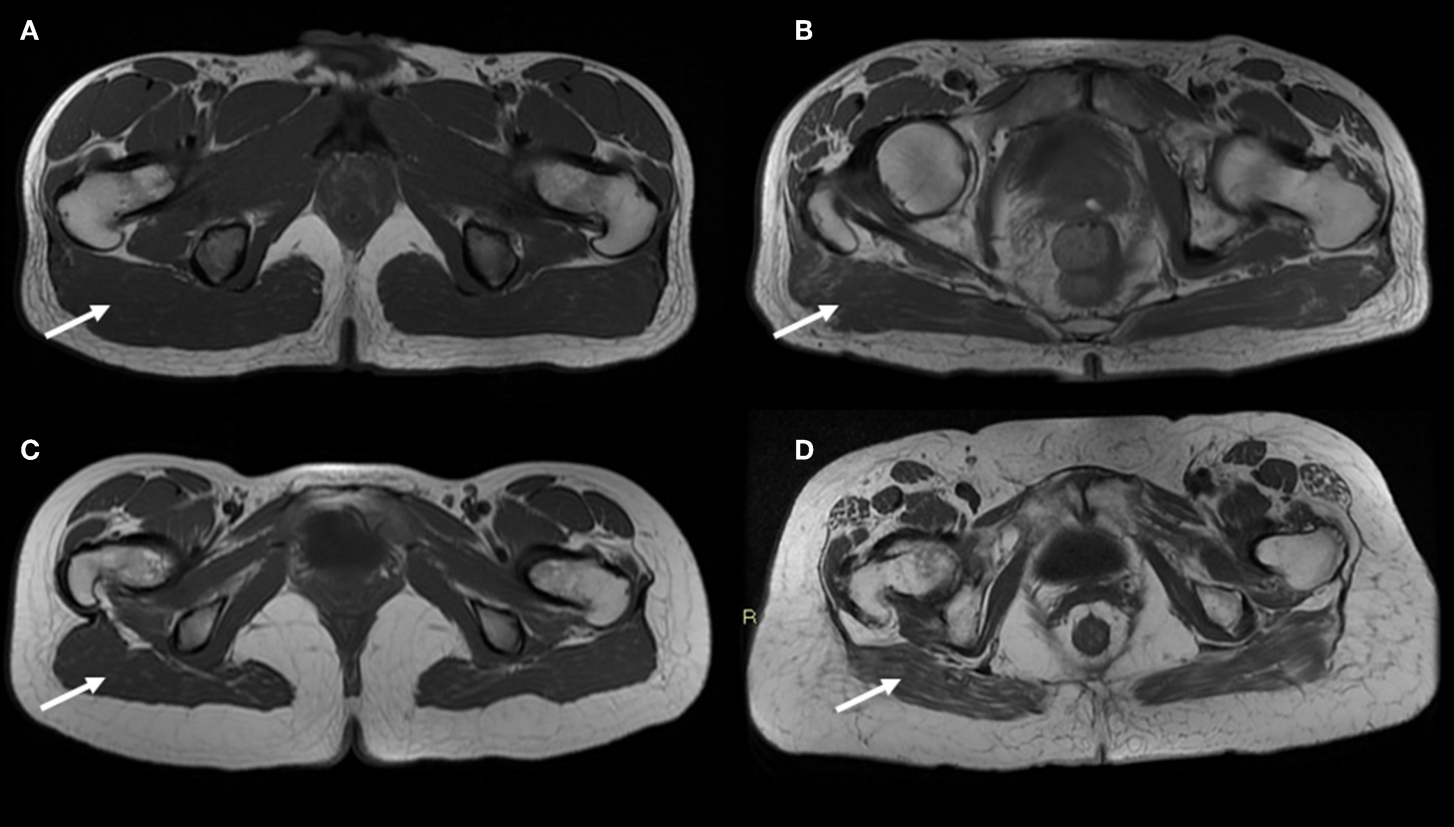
MR T1-weighted image slices of the gynoid region showing age-related muscle changes in both sexes (poor muscle quality and fat infiltration—arrows). In addition larger subcutaneous adipose tissue are observed in the gynoid region of an old female (D) compared to a young female (C); on the contrary the representation of subcutaneous compartment in the same region is the same both for a young male (A) and an old male (B). Images are kindly provided by IRCCS Rizzoli Orthopedic Institute, Unit of Diagnostic and Interventional Radiology (2019).
